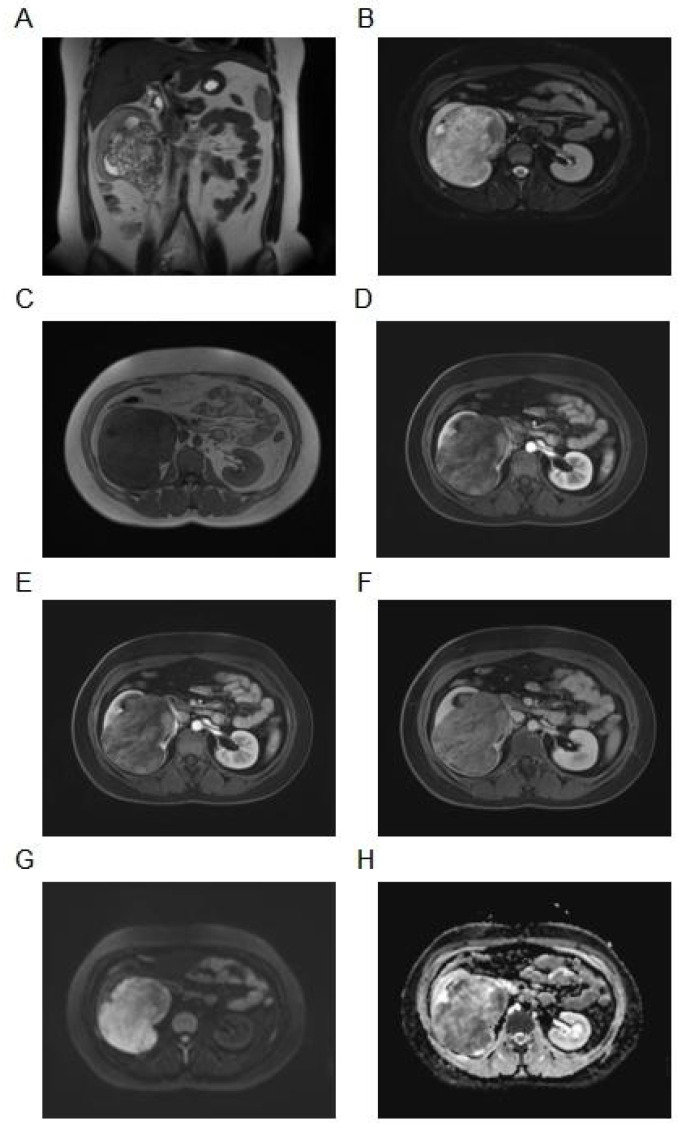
MRI analysis images. (A) Coronal T2-weighted imaging (T2WI): Abnormal high signal intensity within the mass, accompanied by marked dilation of the right renal pelvis (hydronephrosis) and thinning of the overlying renal parenchyma. (B) Axial fat-saturated T2WI: Mixed high and intermediate signal in the mass, with clear compression and thinning of the surrounding renal parenchyma, most evident laterally and superiorly. (C) Axial T1-weighted imaging (T1WI): Predominantly low-to-intermediate signal intensity throughout the lesion. (D) Contrast-enhanced arterial phase: Heterogeneous and uneven enhancement, more pronounced in peripheral zones. (E) Venous phase: Persistent uneven enhancement pattern. (F) Delayed phase: Delayed enhancement of the mass component extending into the proximal ureter, with associated upstream hydronephrosis. (G) Diffusion-weighted imaging (DWI, b=800): High signal intensity in most of the lesion, consistent with restricted diffusion. (H) Apparent diffusion coefficient (ADC) map: Corresponding low ADC values confirming restricted diffusion in the bulk of the mass.
